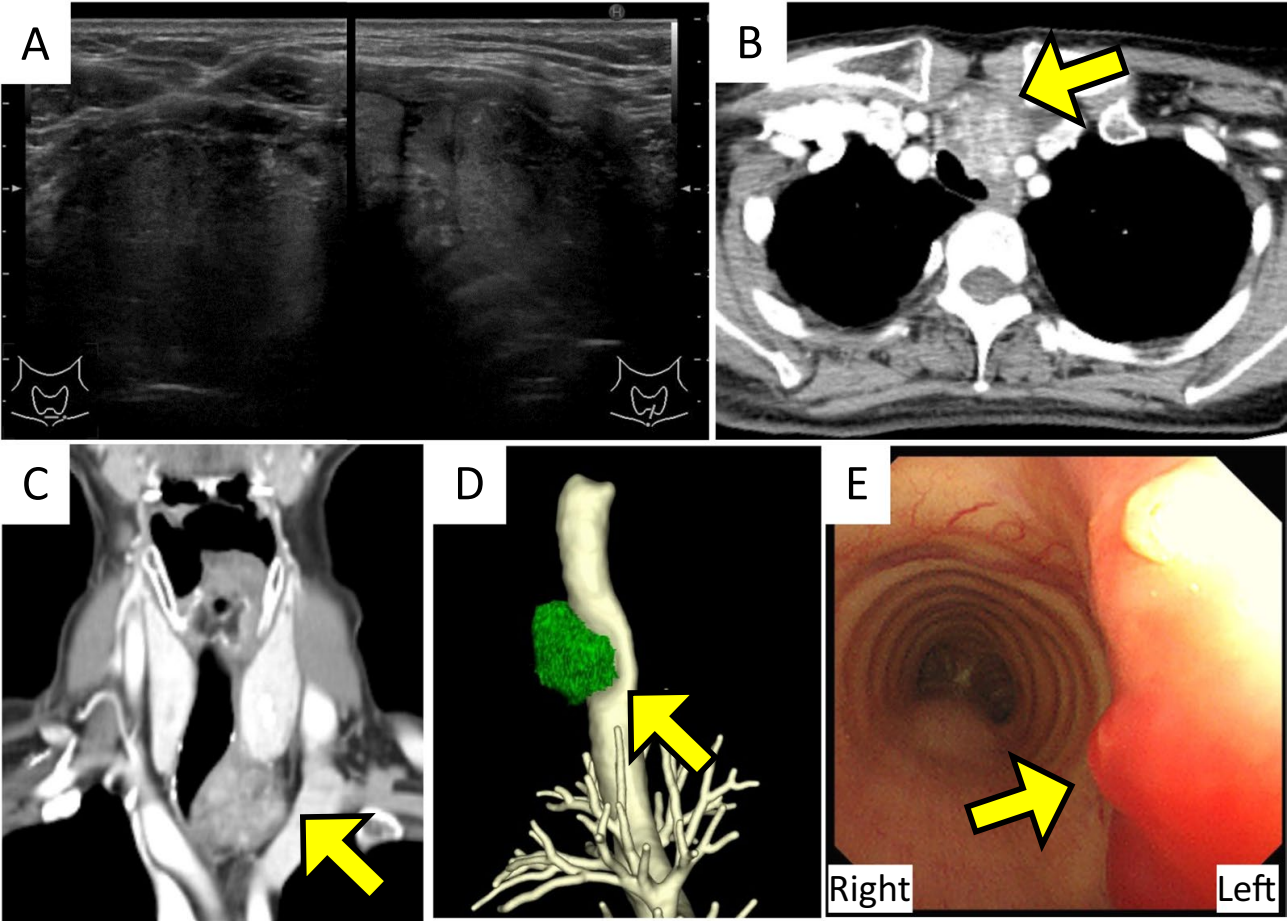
Imaging findings. A Ultrasonographic findings. A hypoechoic mass measuring 34 × 31 mm is observed in the lower pole of left thyroid lobe. B–D Computed tomography findings. A solid mass in the lower pole of left thyroid lobe (arrow) compresses the trachea and partially protrudes into the tracheal lumen, suggesting tracheal invasion. B Axial view, C coronal view, and D provides a 3D reconstructed view. E Bronchoscopic findings. A papillary structure approximately 3 cm in length (arrow) is visible within the tracheal lumen, indicative of tumor exposure
